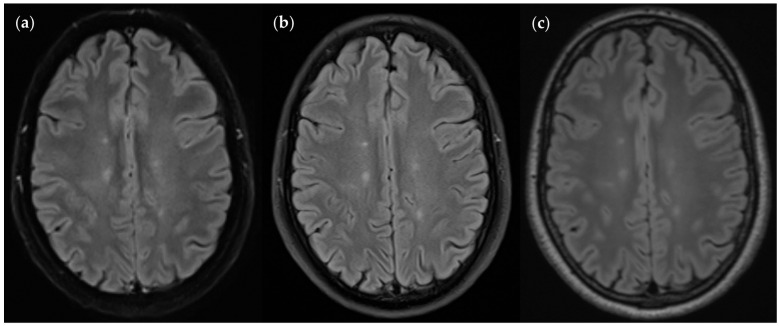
An MS patient received an MRI scan including three T2w FLAIR sequences: FLAIR_UF_ (a), FLAIR_TSE_ (b), and FLAIR_3D_ (c). Five lesions can be seen in each picture, situated in the frontoparietal region.
